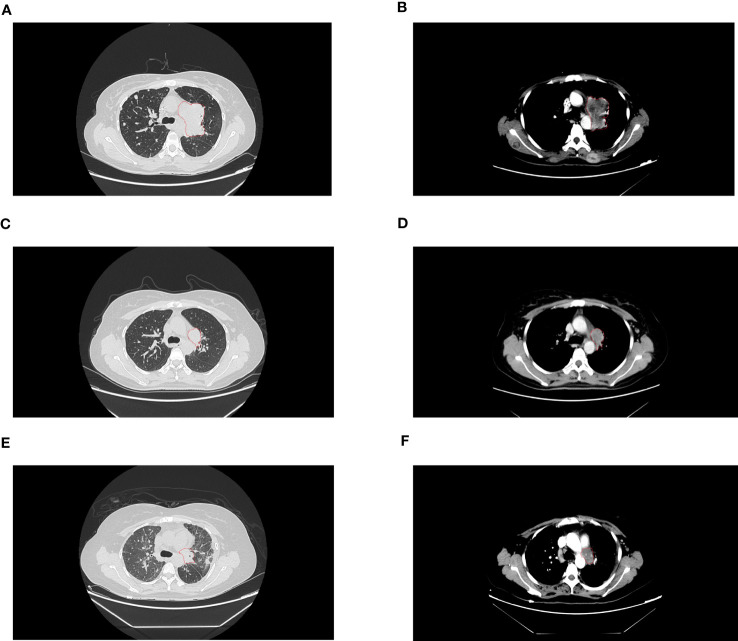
The lesions on computed tomography. (A, B) were before treatment of aletinib. (C, D) were partial response after 8 weeks treatment of aletinib. (E, F) were progressive disease after 11 months treatment of *ALK*-TKI.
